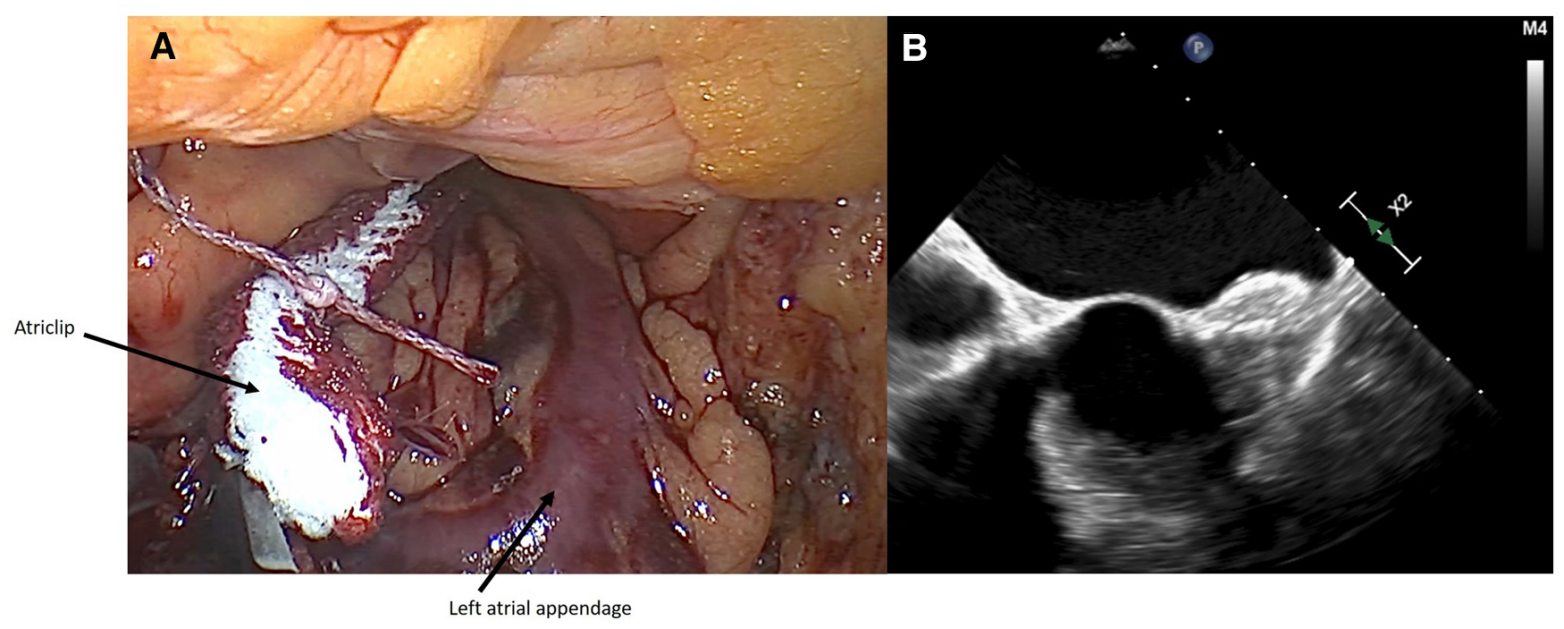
(A) intraoperative closure of LAA with AtriClip PRO2® device. (B) Intraoperative transesophageal echocardiography showing successful closure of LAA.
